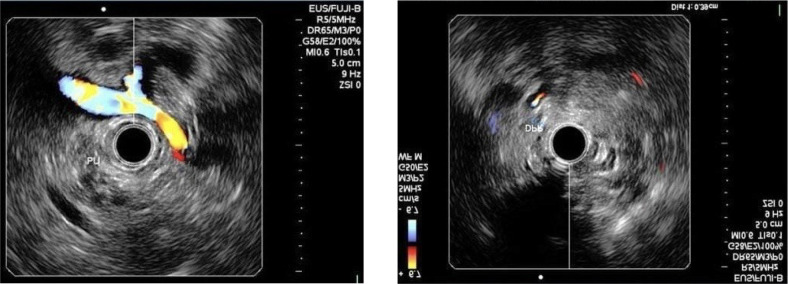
Endoscopic ultrasound confirming *pancreas divisum.*
